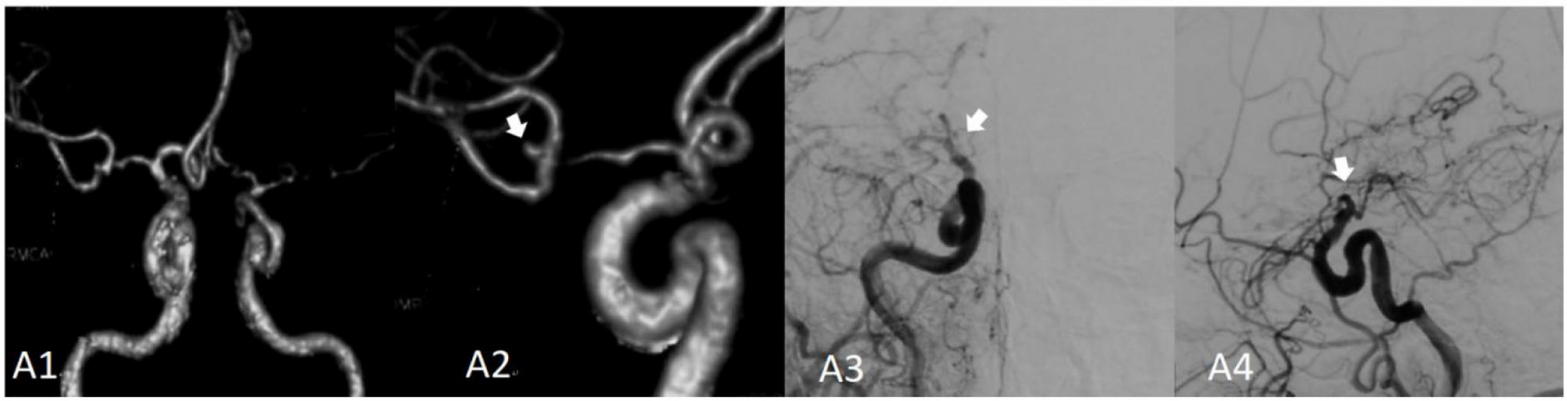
Moyamoya disease combined with a bifurcation aneurysm of the right middle cerebral artery. (A1) Shows the computed tomography angiography (CTA) image of the bilateral internal carotid artery in 2014; (A2) shows the locally enlarged CTA of the right internal carotid artery; the aneurysm is indicated by the white arrow. (A3) Presents the right internal carotid artery angiography (red arrow) image. (A4) Presents the right internal carotid artery angiography image; the distal end of the internal carotid artery is not occluded, and the parent artery and aneurysm have disappeared.
